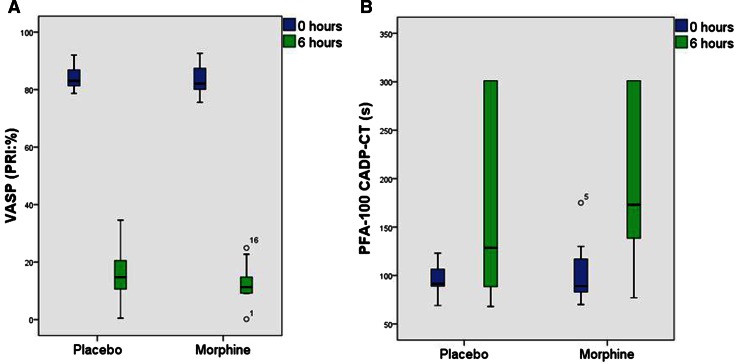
Morphine does not blunt prasugrel effects. *Box- and whisker plot* of the vasodilator-stimulated phosphoprotein (VASP) phosphorylation (a) and the collagen adenosine diphosphate closure time (CADP-CT) (b) 0 and 6 h after co-administration of prasugrel and placebo/morphine. VASP phosphorylation was measured by flow-cytometry (*n* = 12) and the CADP-CT with the platelet function analyzer (*n* = 12). Data present medians with 25th and 75th percentiles (minimum and maximum)
